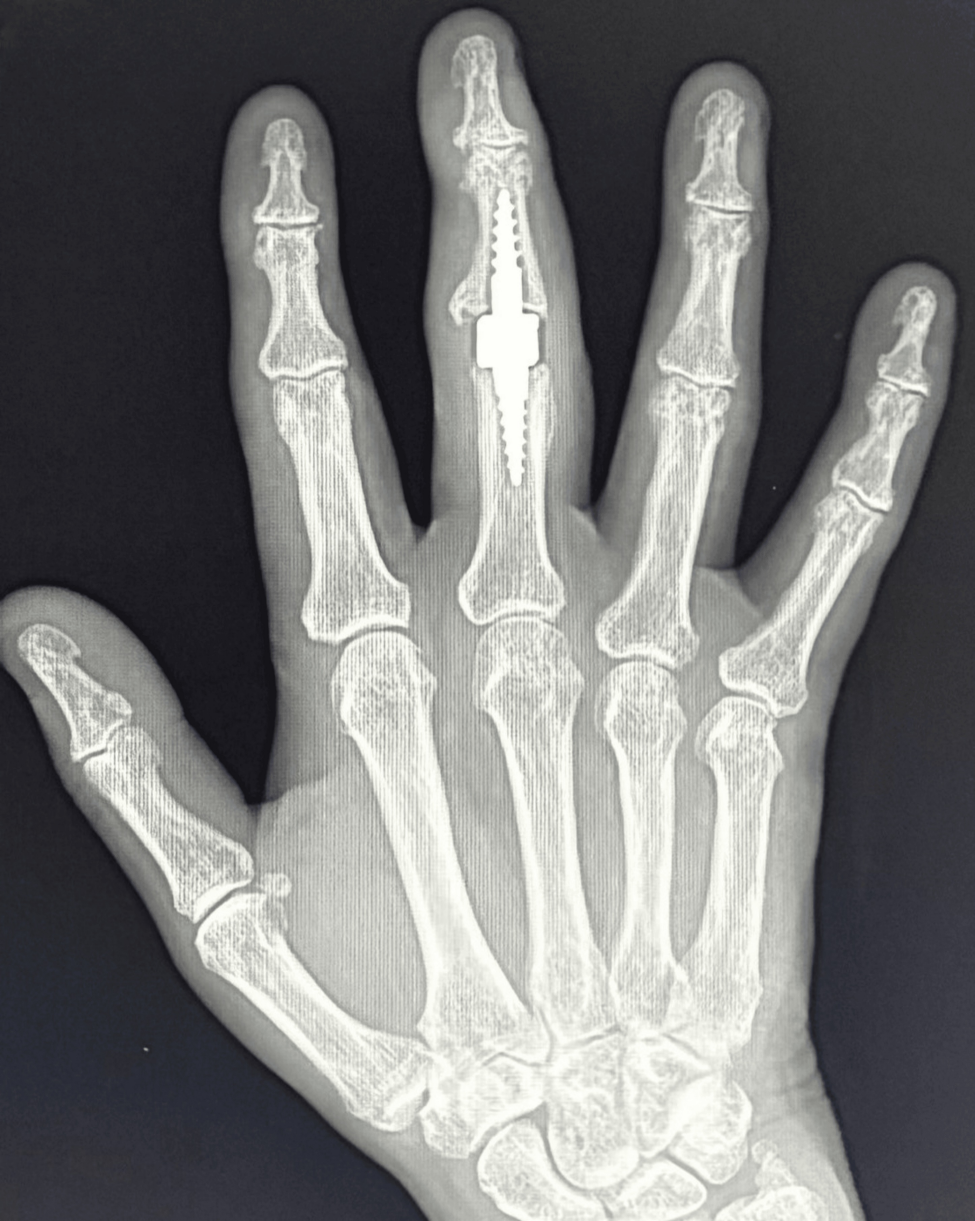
Control X-ray in AP projection six month after surgery
